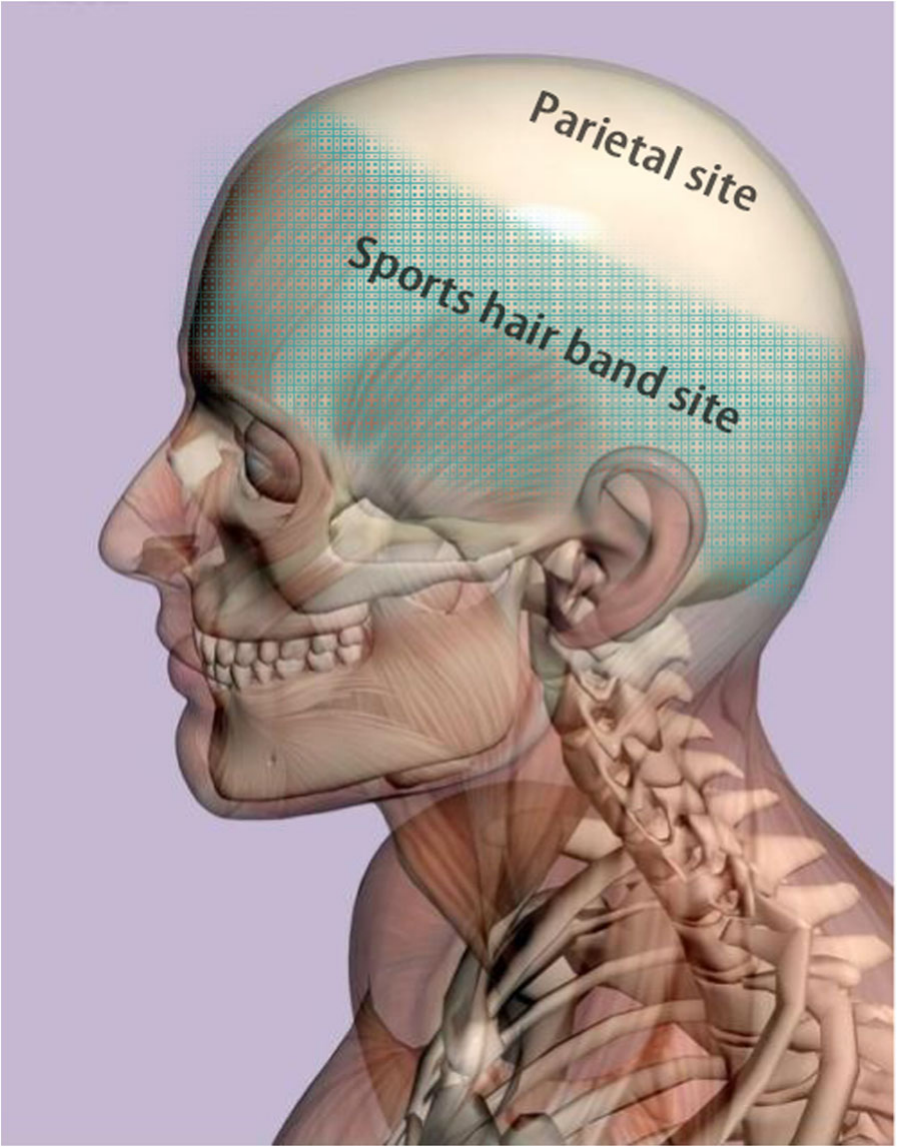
The line and the two sites. This supposed line, which is shown with a green and yellow border line, includes the bilateral temporal lines, coronal suture, and parietooccipital sulcus in the sagittal MRI. The upper part was near the parietal lobe, with little muscle attached. As the midline of the dura is not easy to watertight suture, little muscle is covered, and it is difficult to apply a compression bandage. Therefore, we named it “parietal site”, which is shown in the figure. The subdural CSF can more easily flow to the subcutaneous tissue and form SFC and not STH. The lower part is far away from the top of the head and with thick muscle attachment, which could be compression bandaged. It is similar to having a sporting hairband on your head. Therefore, we named it “Sports hair band site” in this study, which is shown in figure with green colour. The subdural fluid has difficulty flowing through the dura and muscle to the subcutaneous tissue. This means that it is easier to form STH than SFC
